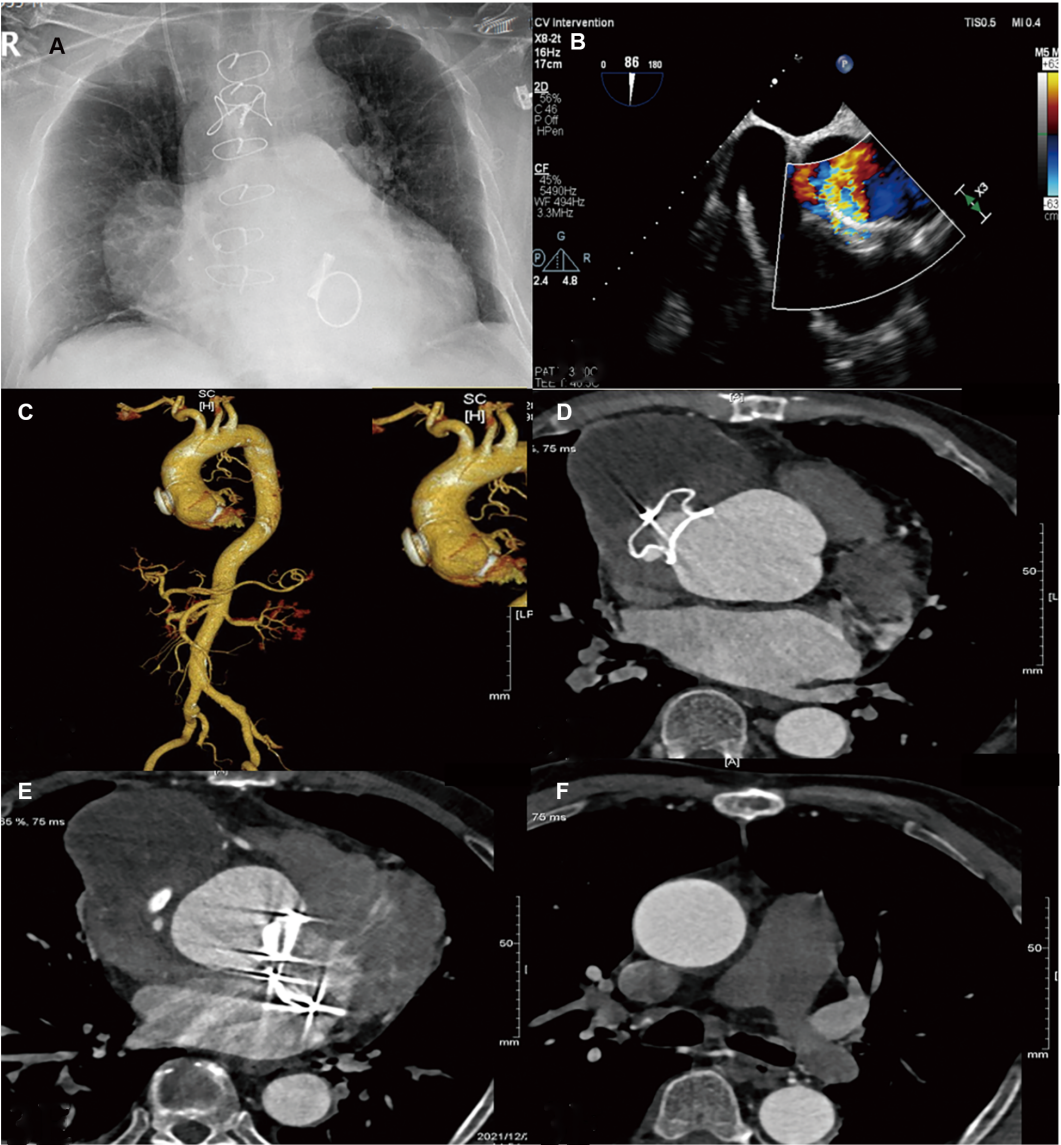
Preoperative: (A) Chest ray; (B) Cardiac ultrasound; (C–F) CT of the coronary artery + thoracic and abdominal aorta [(C) Front position; (D) Tumor waist; (E) Lower border of the tumor; (F) Pulmonary bifurcation)].
